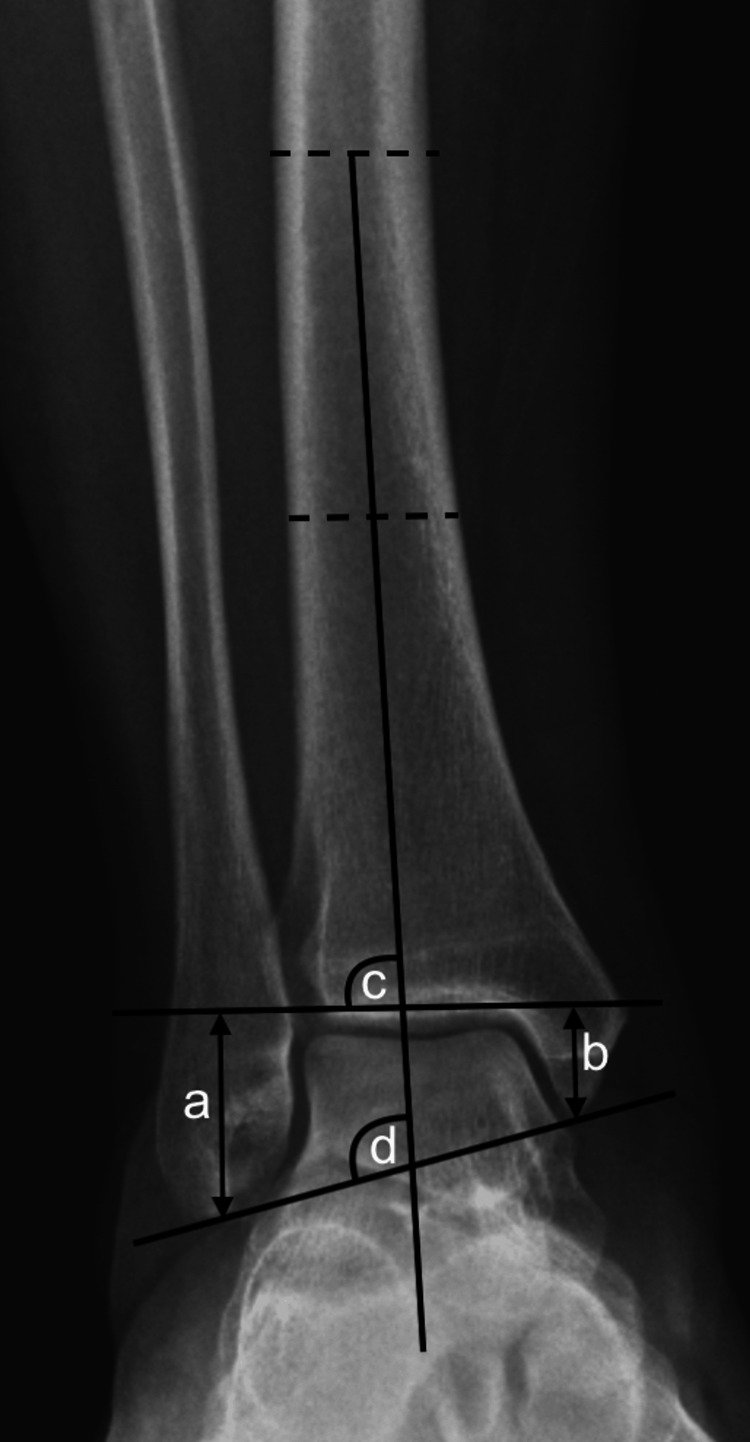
Measurements evaluated on anteroposterior ankle radiographs. a. Length of the lateral malleolus, b. Length of the medial malleolus, c. Distal tibial articular surface angle, d. Bimalleolar tilt.
